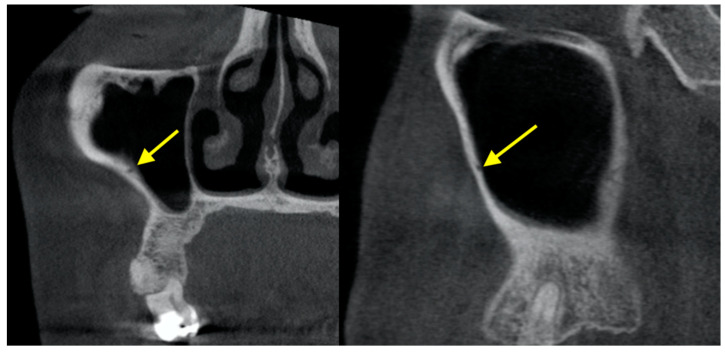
Posterior superior alveolar artery shown in CBCT.
